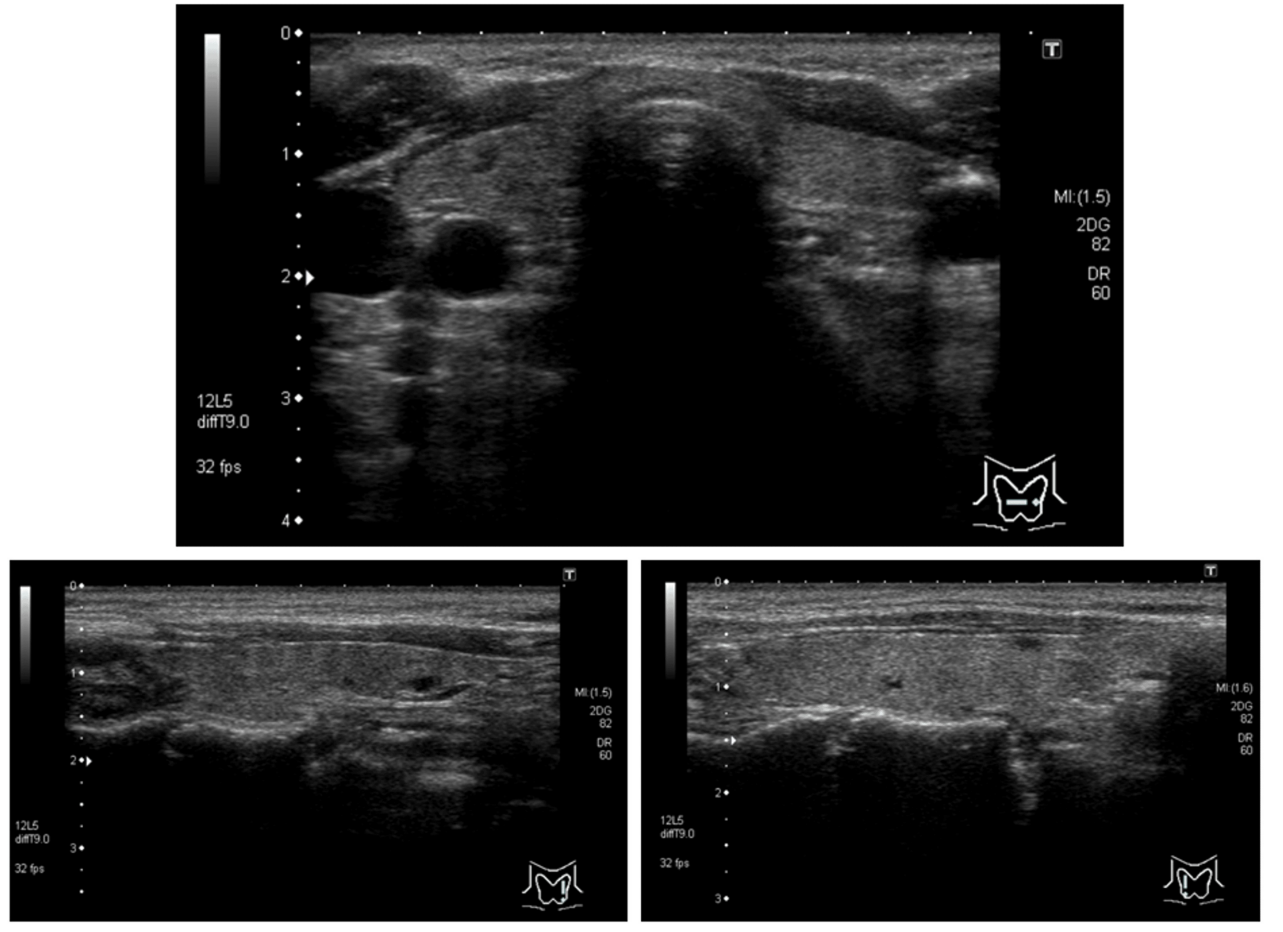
Thyroid ultrasound image No enlarged parathyroid glands were observed.
